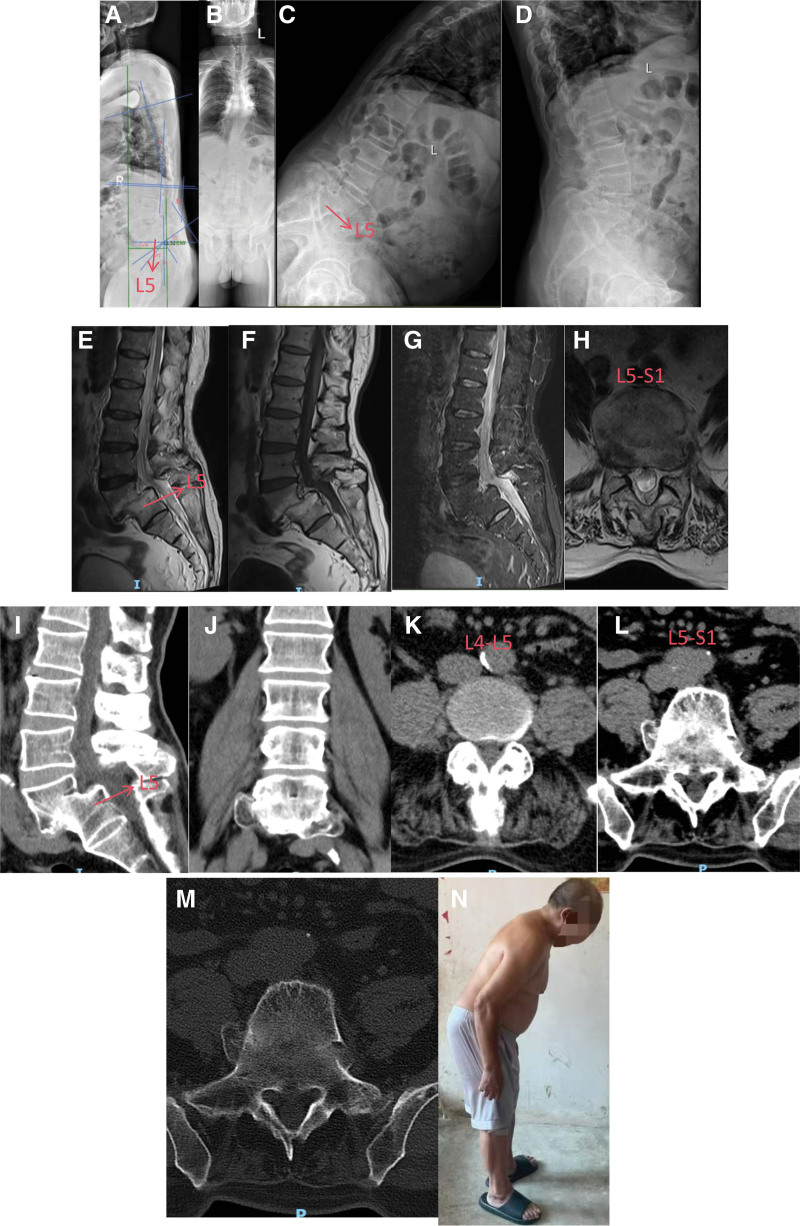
(A and B) Radiographs demonstrate lumbosacral spondylolisthesis with a lumbosacral block vertebra; SVA = 11.52 cm; LL = 39°; TK = 18°; pelvic parameters: PI = 75°; PT = 40°; SS = 35° – indicative of severe sagittal imbalance. (C and D) Flexion–extension lumbar radiographs reveal partial L4 to 5 fusion with stable alignment. (E–H) MRI shows grade II anterolisthesis of L4 with obliteration of the L4 to 5 disc space. (I–M) CT shows: (1) L3–4 disc bulging with mild protrusion and mild canal stenosis at L3–4 and L4 to 5. (2) Bilateral L4 pars interarticularis defects with grade II anterolisthesis and partial L4 to 5 fusion. (N) Demonstrates ~40° anterior trunk inclination in the sagittal plane. (two independent spine surgeons performed blinded, repeated measurements with concordant results).
